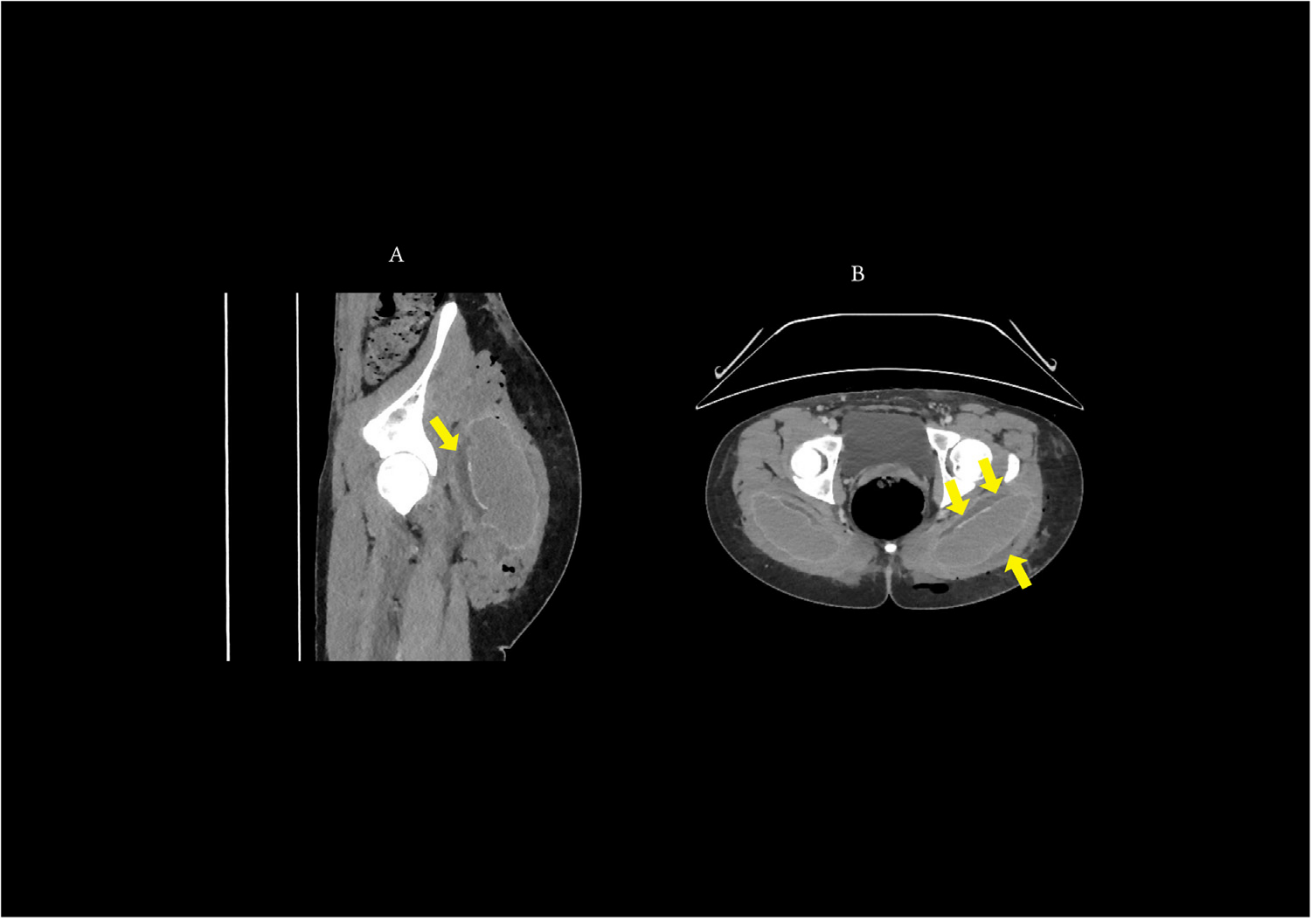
Computed tomography (CT) findings in postoperative gluteal infection. A: Sagittal CT image of the left buttock showing a periprosthetic fluid collection surrounding the silicone implant (yellow arrow) within the intramuscular plane of the gluteus maximus, with adjacent gas locules suggestive of infection. B: Axial CT image demonstrating bilateral periprosthetic fluid collections (yellow arrows) and subcutaneous emphysema, more pronounced on the left side, consistent with severe postoperative infection.
